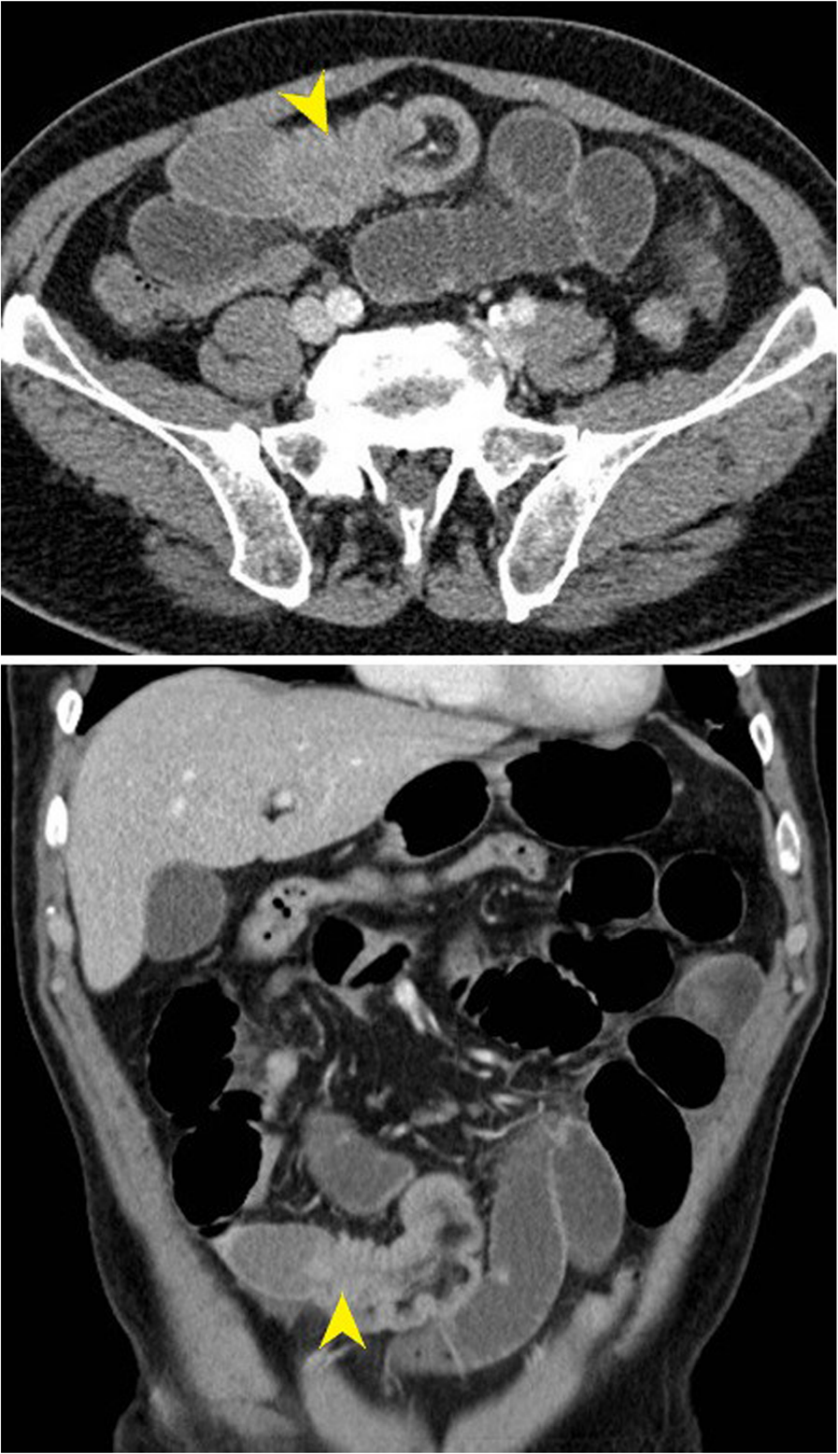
Emergency computed tomography scan demonstrating small bowel intussusception with a suspected intraluminal lesion as a lead point (*arrows*)
